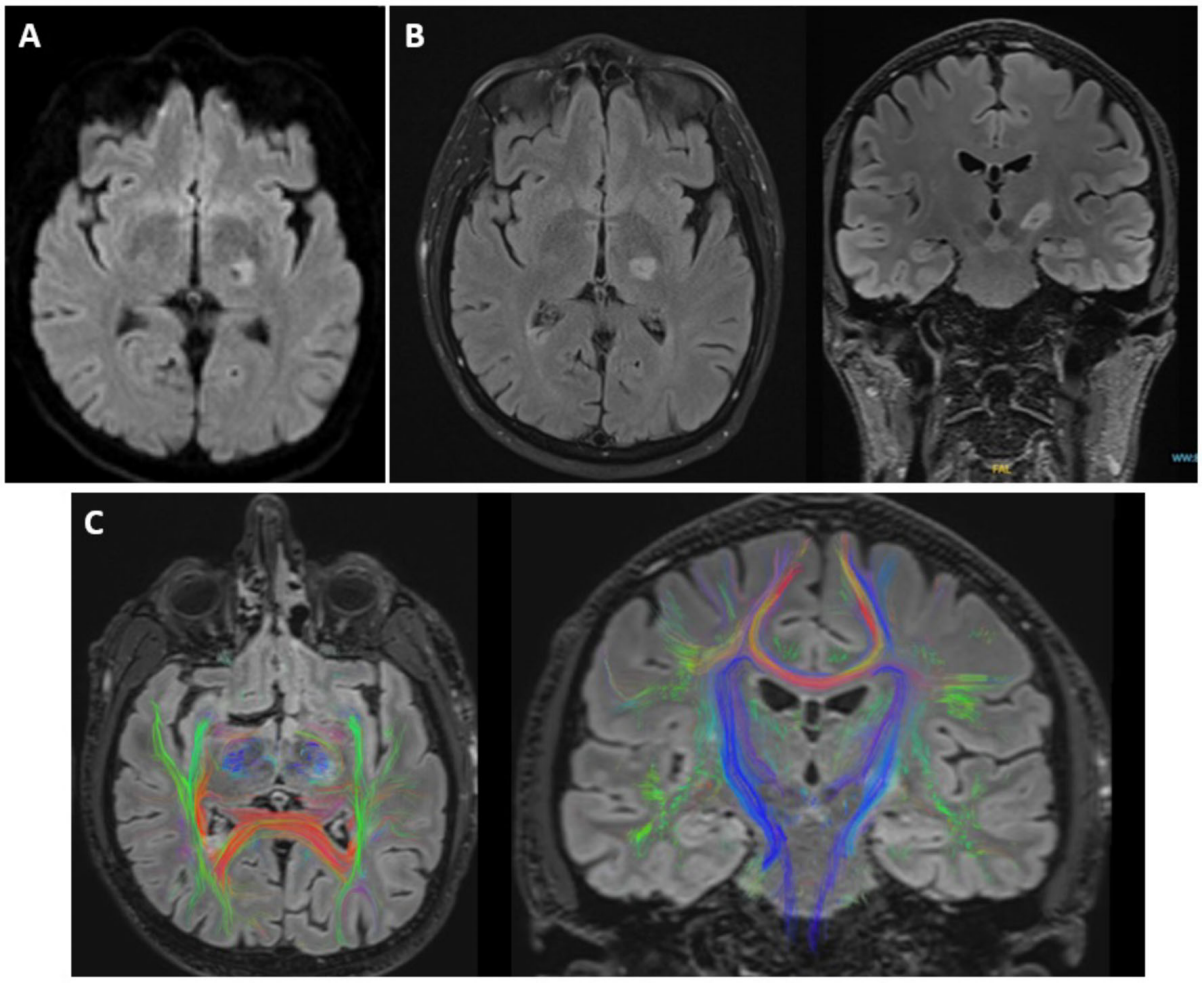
Brain MRI with diffusion‐weighted imaging (DWI) (A) and fluid‐attenuated inversion recovery (FLAIR) (B) shows a recent ischemic lesion in the left internal capsule and thalamus. Fiber tractography (C) reveals impairment of the pyramidal tract (in blue).
